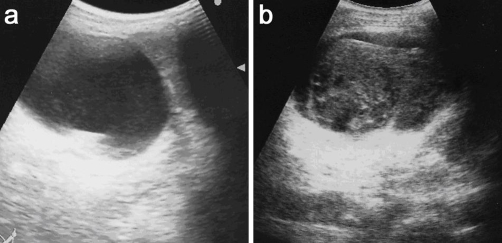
Ultrasonography examination revealed heterogeneous mass in the cyst (b), which had not been found 3 days before (a).
